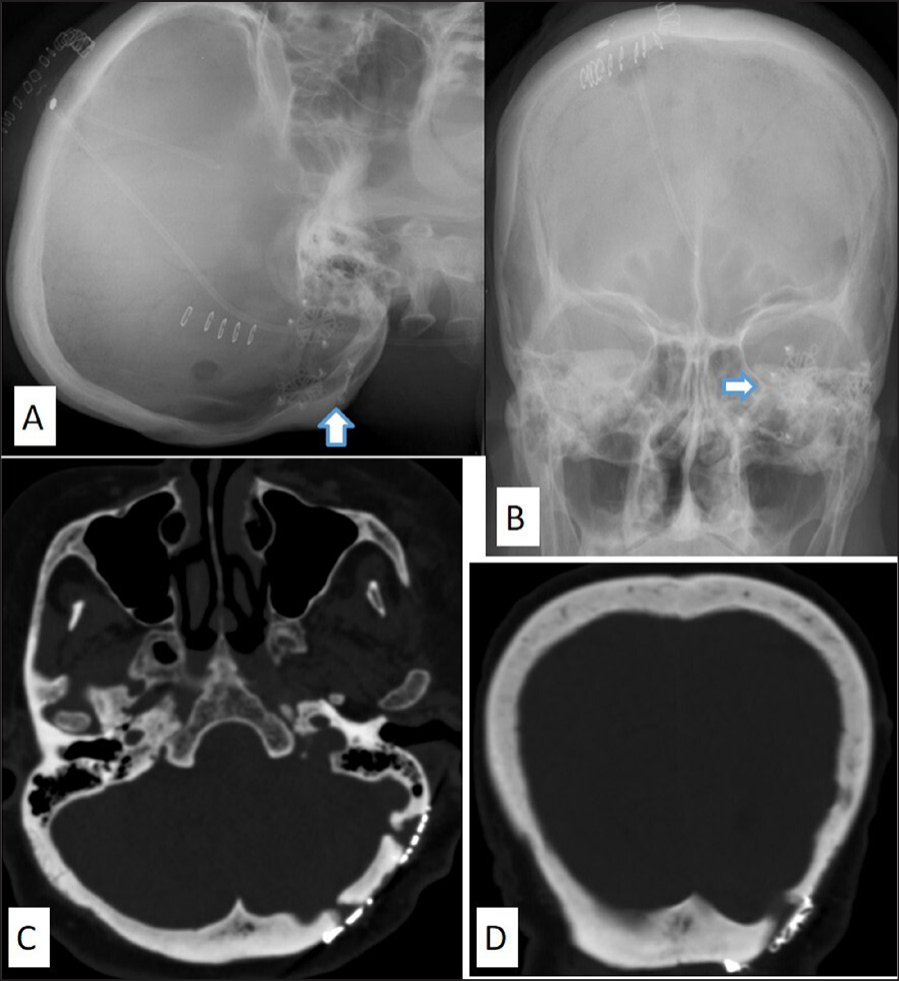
Lateral and anteroposterior skull radiographs (A) and (B) a 55-year-old man who underwent craniotomy shows two metallic cover plates or craniofix (Aesculap) on the left side of the posterior fossa (A, arrows). Axial CT on skull window (C) and (D) of the same man shows the metallic cover plates.
